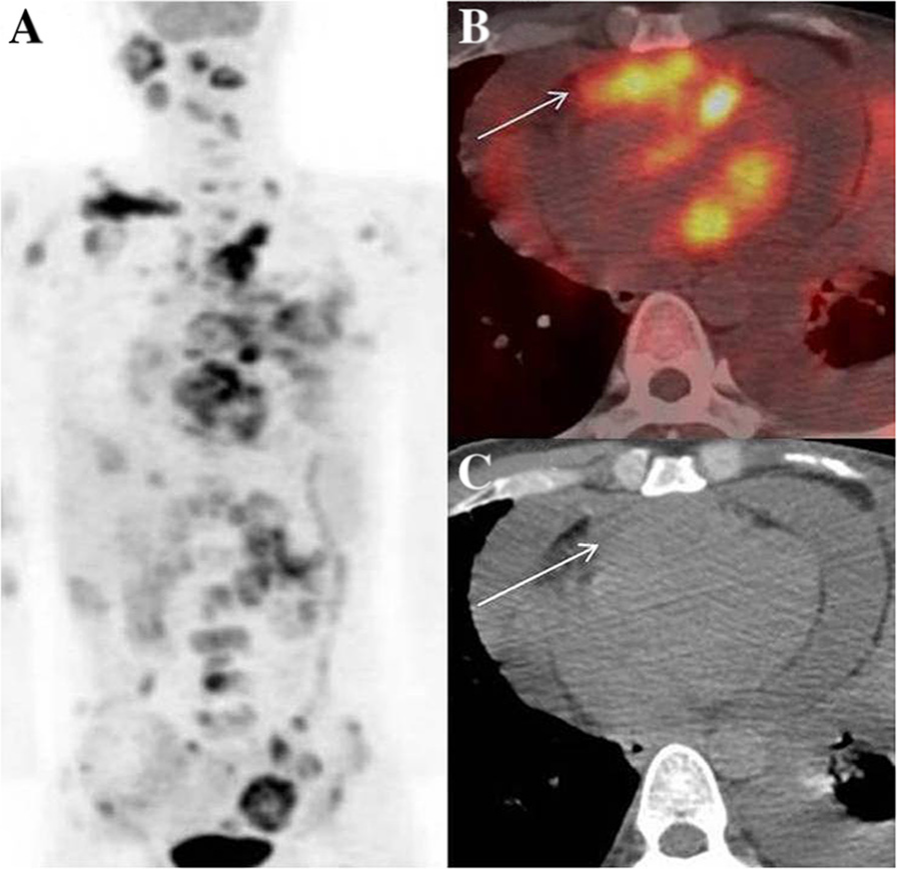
Myocardial metastases from buccal mucosal cancer. A - MIP image showing multiple metastatic foci, B- Axial fused PET/CT image showing tracer uptake in the cardiac region (arrow), which corresponds to C -hypodense lesion on axial CT image (arrow).
